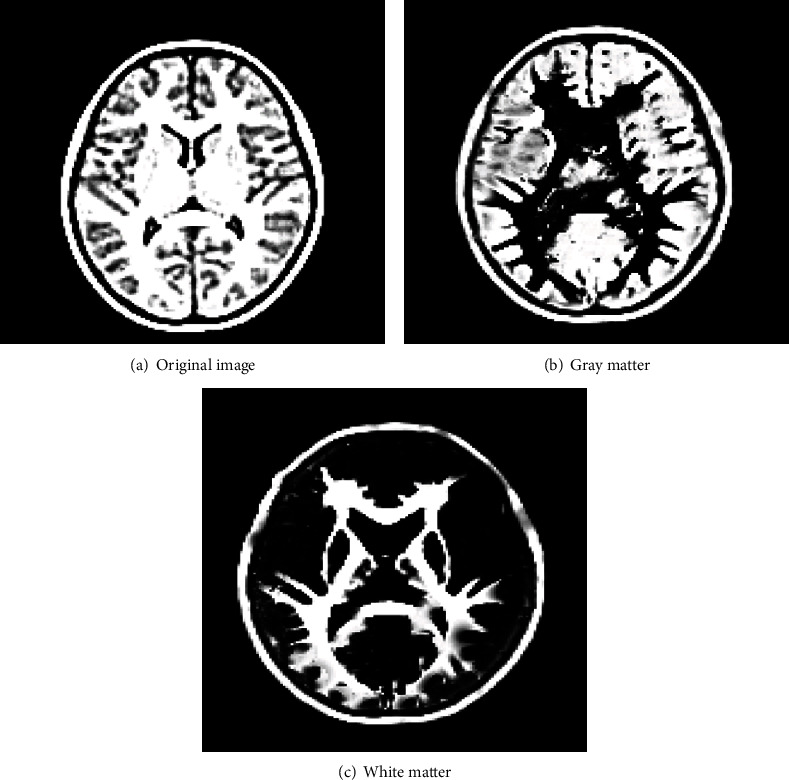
MR images of FCM algorithm brain.
